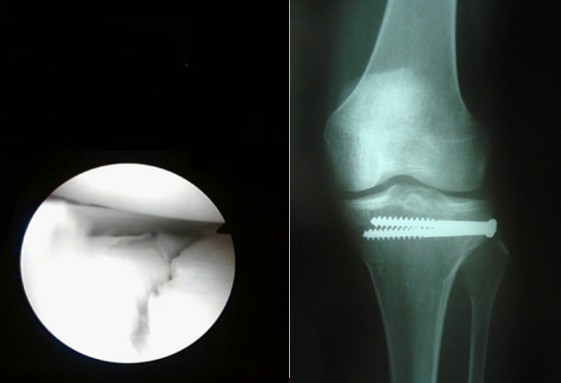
A: vue arthroscopique de réduction de séparation pure; B: radiographie de controle après vissage
